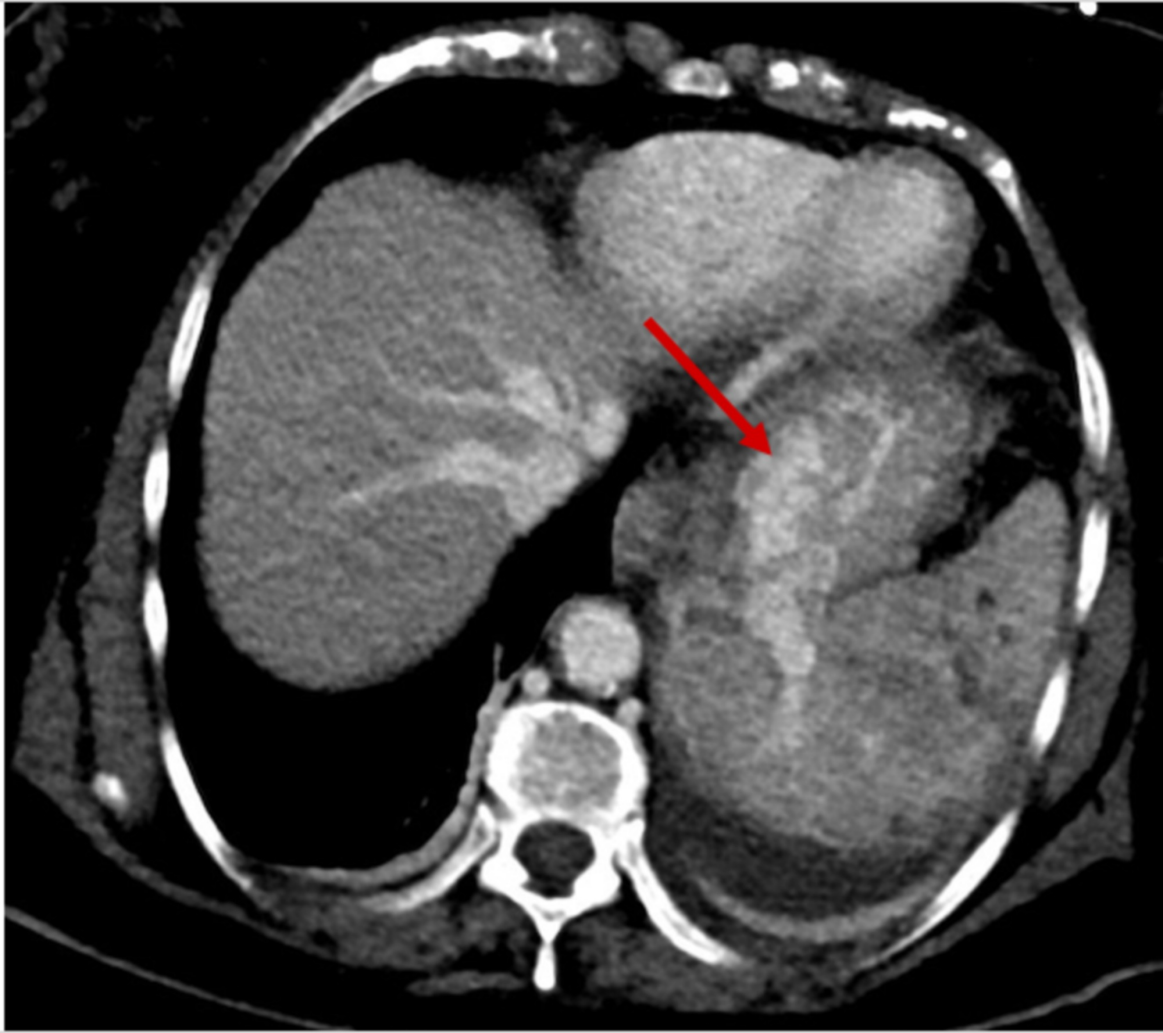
Axial abdominal computed tomography (CT) scan Portovenous delay showing gastric fundal varices (red arrow)
